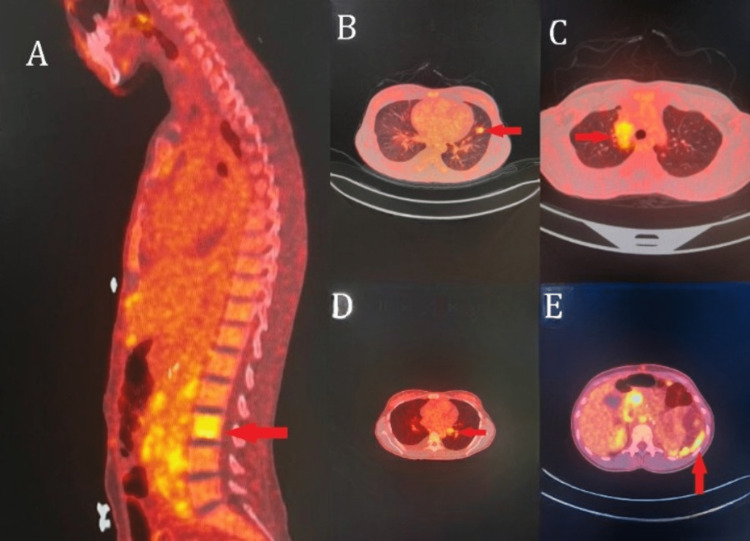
PET/CT Scan Five Weeks After ICI Treatment. (A) Evidence of a new area with abnormally increased FDG uptake involving the left aspect of L3 lumbar vertebrae. (B) New multiple bilateral lung nodules, variable in size, showing a variable degree of abnormal increased FDG uptake being more prominent in the upper lobe of left lung SUV max 3.4. (C) New mediastinal lymph node involvement showing abnormal increased FDG uptake. (D). New left hilar lymph node showing abnormal increased FDG uptake. (E) Evidence of new multiple peritoneal soft tissue densities showing abnormal increased FDG uptake. ICI: immune checkpoint inhibitor; FDG: fludeoxyglucose; SUV: standardized uptake value
